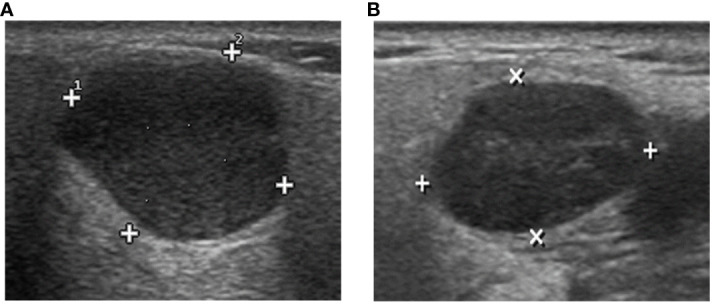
The DL algorithm correctly classified (A) biopsy-PA nodule with a WT appearance as PA and (B) biopsy-WT nodule with a PA appearance as WT. However, the sonographic features demonstrate similarities in the images, making it difficult to classify the two tumors by ultrasound physicians.
